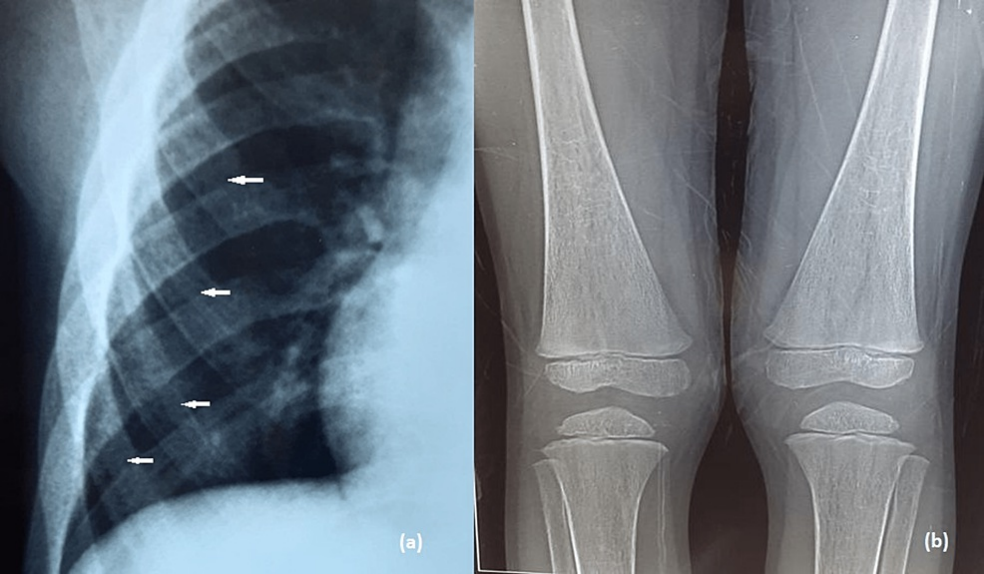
Magnified view of chest radiograph PA view (a) showing expansion of ribs with a “rib-within-a-rib” appearance, particularly in the anterior portion (white arrows). Knee radiograph (b) showing bilateral metaphyseal flaring with Erlenmeyer flask deformity.
